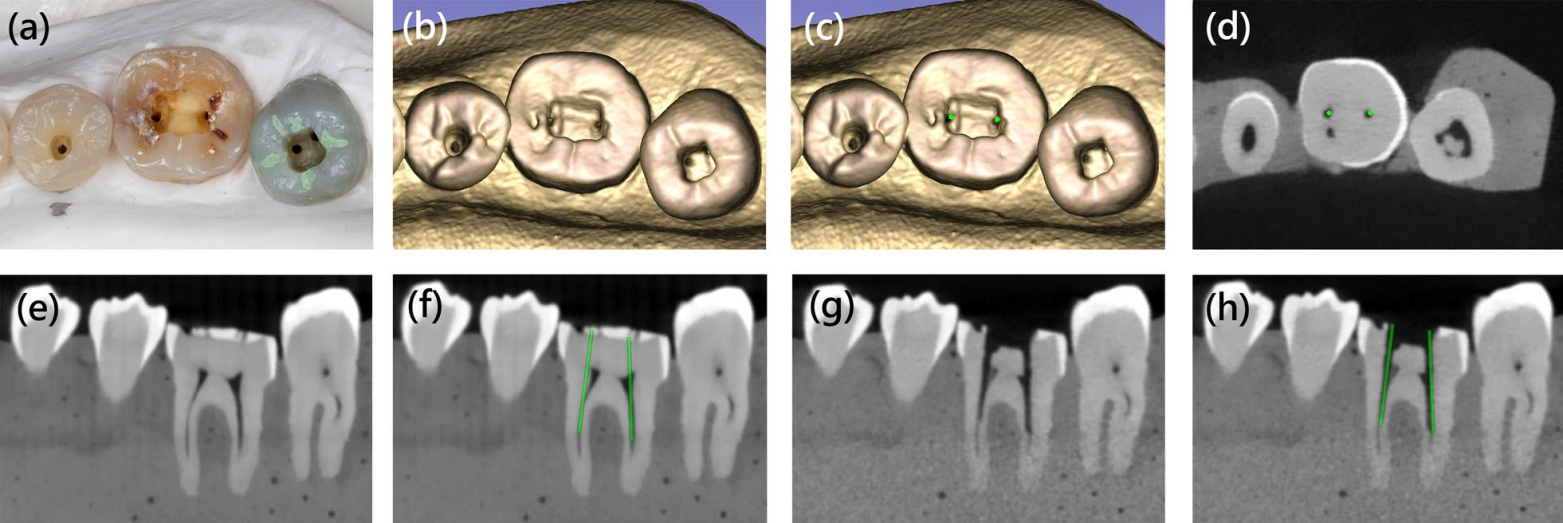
Superimposition of clinical, preoperative, and postoperative images: a Clinical photograph of right lower first molar after enamel removal and template drilling. b 3D reconstruction of postoperative cone beam computed tomography (CBCT). c Virtual image of burs (green cylinder) was superimposed on 3D reconstruction of postoperative CBCT. d Axial view of postoperative CBCT, the vital burs (green dot) overlapped with the paths of drills. e Sagittal view of preoperative CBCT. f Sagittal view of preoperative CBCT with virtual burs (green cylinder). g Sagittal view of postoperative CBCT, and the paths of drills penetrated into mesiolingual and distal canal. h Sagittal view of postoperative CBCT with virtual burs (green cylinder). Slight deviation was noted between virtual burs and the paths of drills
